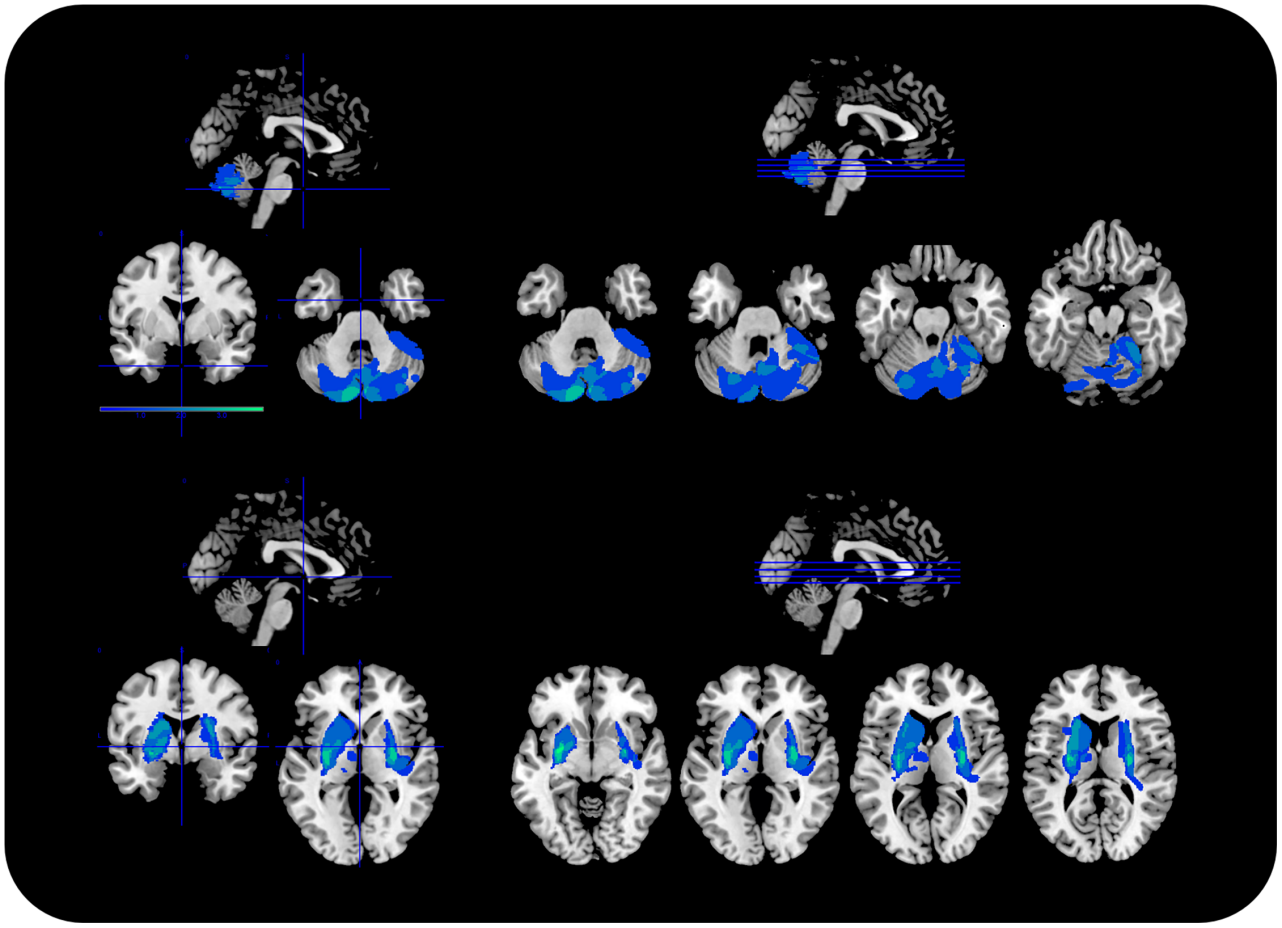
Brain lesion delineation and overlap. The figure provides the brain lesion delineation on a template anatomical MRI for cerebellar (CE; top) and basal ganglia (BG; bottom) patients. The figures were obtained by using MRIcron (http://www.mccauslandcenter.sc.edu/mricro/mricron/), and display the lesion overlap color-coded in shades of blue (blue = minimal overlap; light blue high overlap; values range from 0 to 5).
